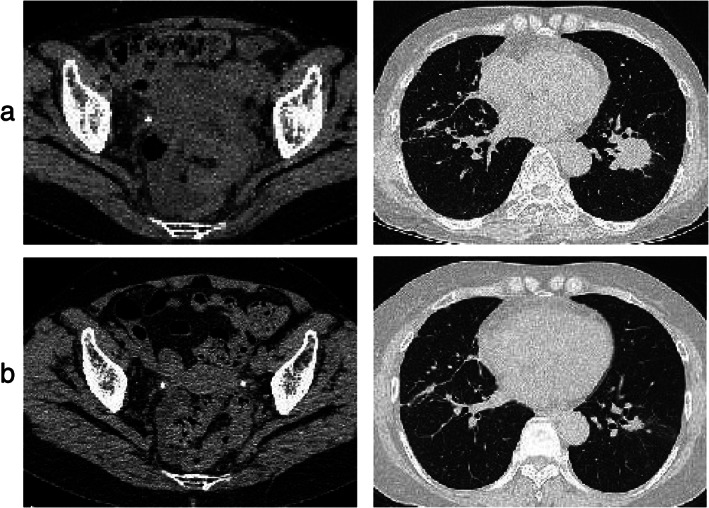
Lower abdominal and chest computed tomography findings before (a) and after (b) treatment with ceritinib in the present case
